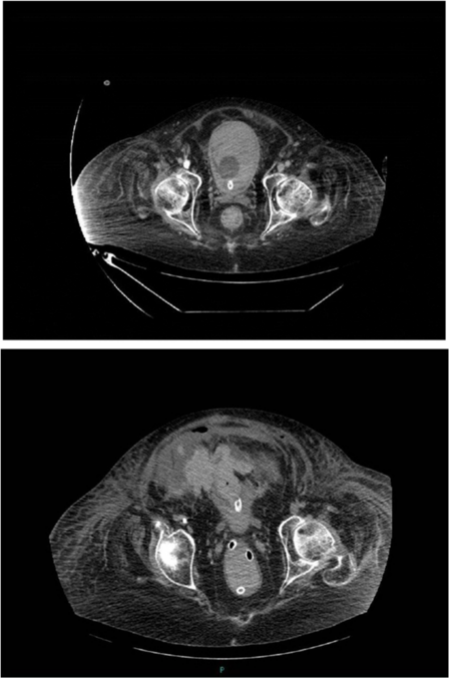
Top panel: Axial section of CT of abdomen performed five days after vesical lithotripsy: A 8
